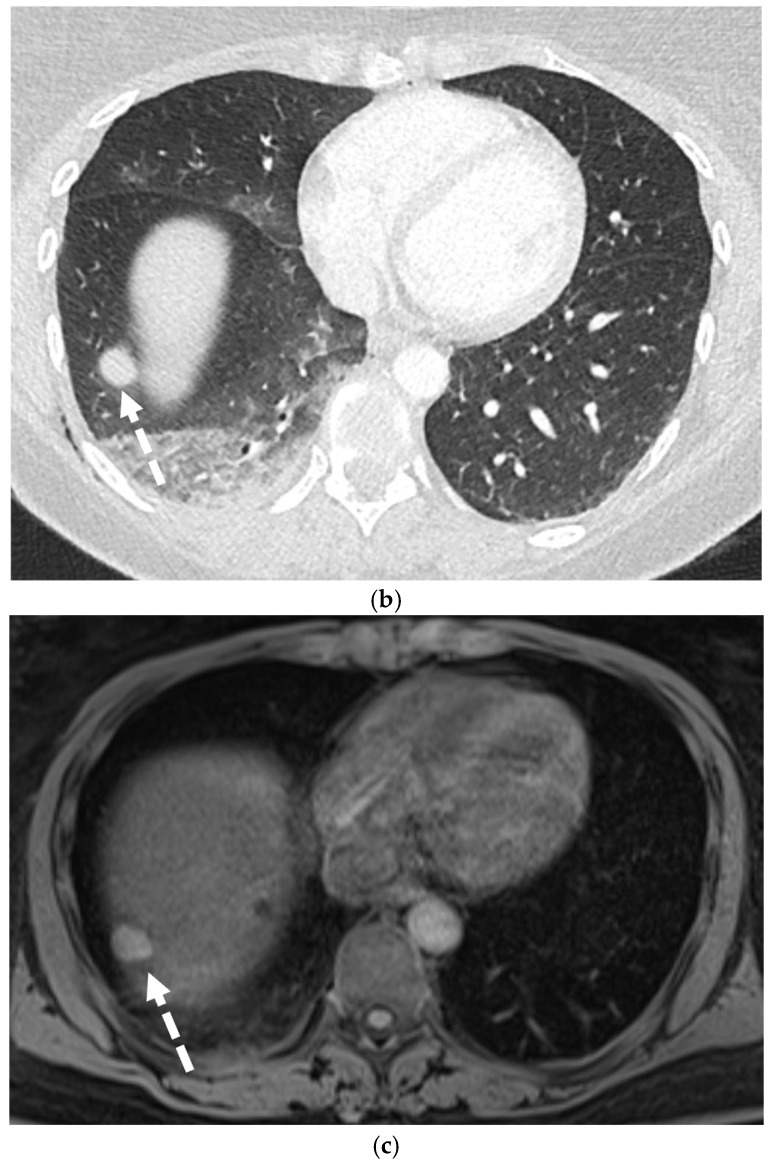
42-year-old female presents with right-sided chest pain. Frontal chest radiograph (a) demonstrates large right pneumothorax (white star). Axial CT image in lung window performed after placement of right pleural drain (b) shows patchy ground-glass opacities in right-lower lobe and solid nodule (dashed white arrow) along right hemidiaphragm. Axial fat-suppressed T1W MR image (c) shows high signal intensity of solid nodule (dashed white arrow) along right hemidiaphragm indicating subacute hemorrhagic/proteinaceous content. These findings are compatible with endometriotic implants with catamenial pneumothorax.
